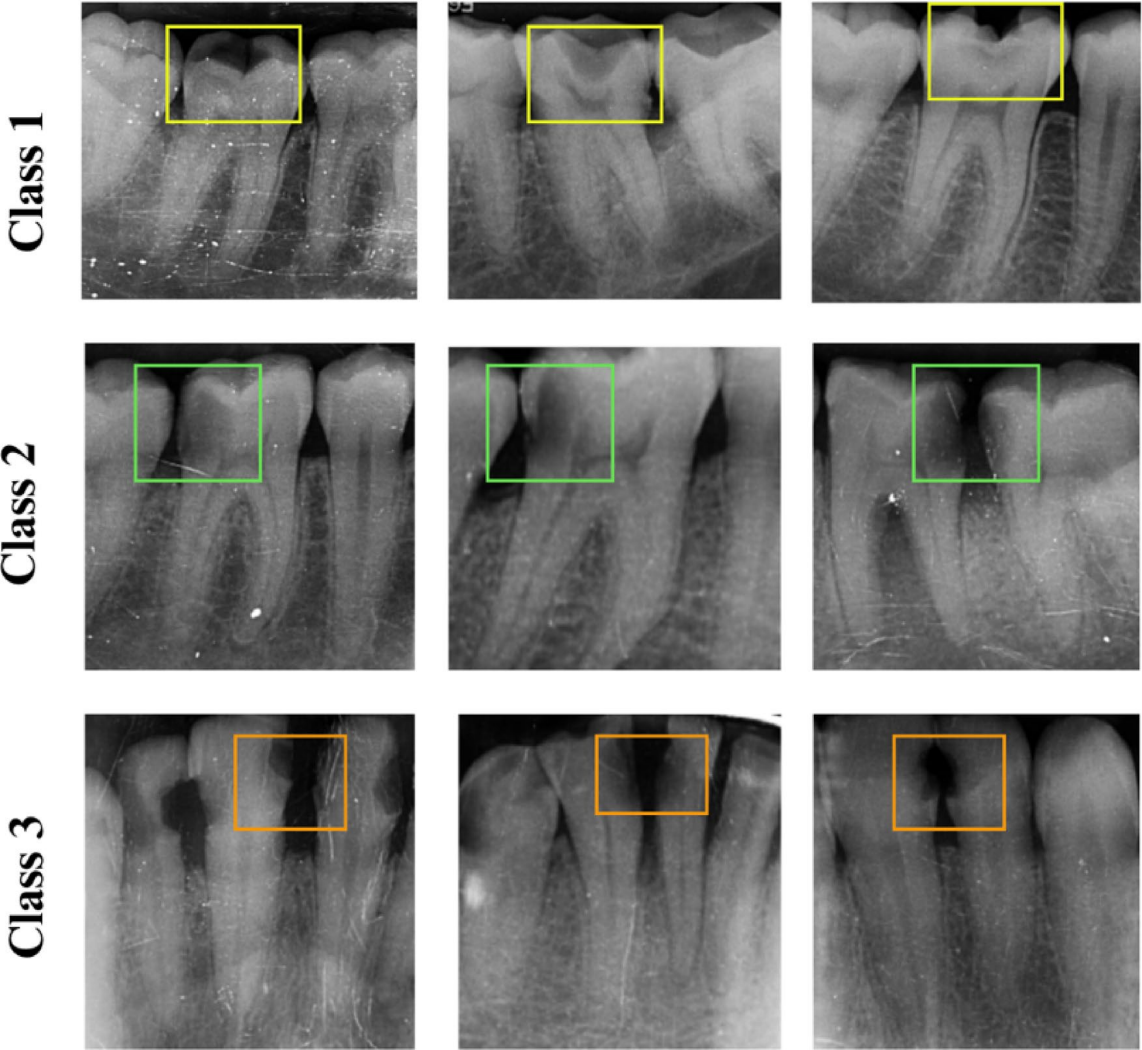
Sample intraoral periapical radiographs depicting G.V. Black Class I–III caries, with carious regions highlighted by bounding boxes.
